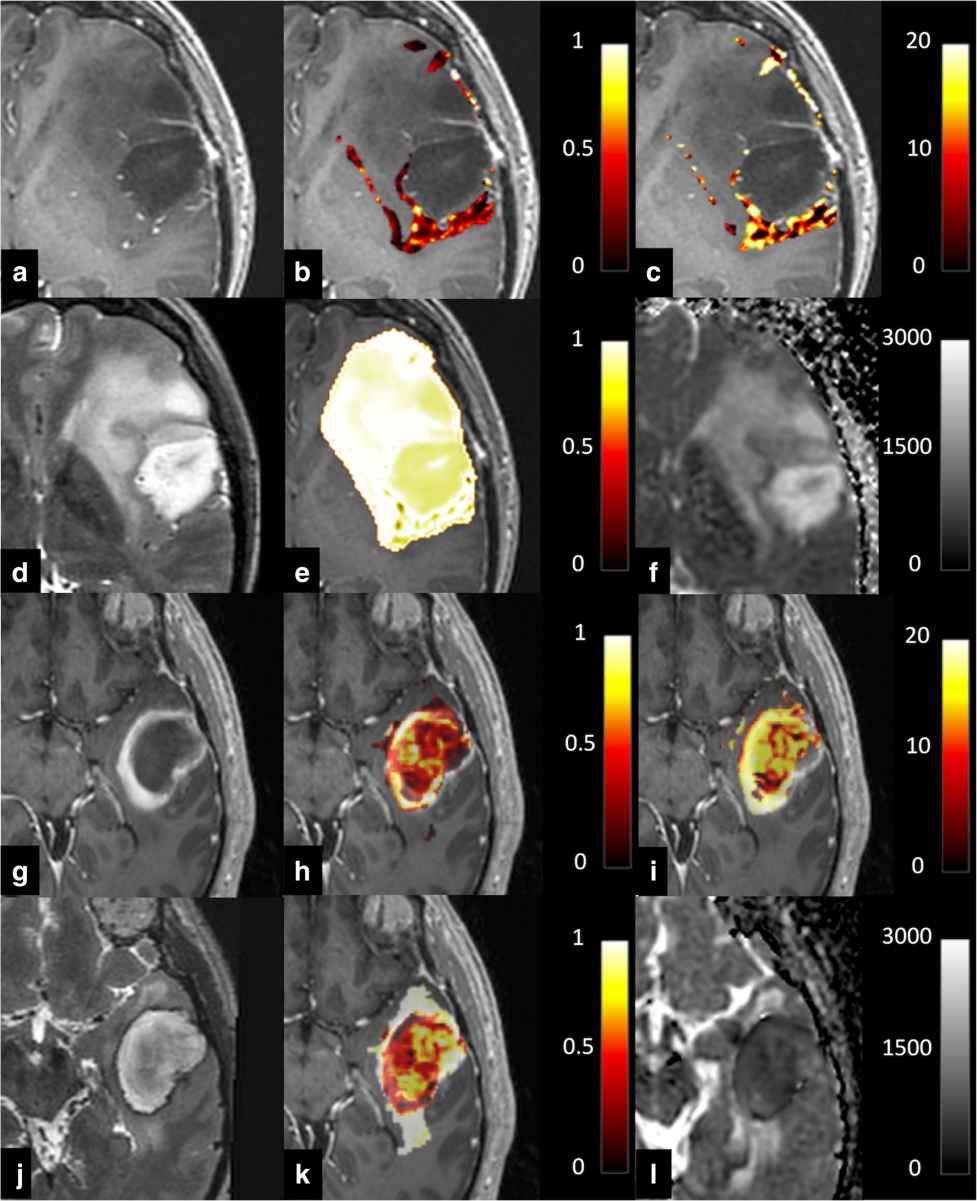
a–f Representative images from a low-grade glioma. a Axial post-gadolinium 3D T1-weighted imaging (T1WI). b Intracellular volume fraction. c Cell radius maps. d Axial T2WI. e Extracellular volume fraction. f ADC map, with a scale of × 10^−6^ mm^2^/s. Colour maps for b, c, e have been superimposed on the greyscale image from a with the colour scale shown for each image. g–l Representative images from a high-grade glioma. g Axial post-gadolinium 3D T1WI. h Intracellular volume fraction. i Cell radius maps. j Axial T2WI. k Extracellular volume fraction. l ADC map, with a scale of × 10^−6^ mm^2^/s. Colour maps for h, i, k have been superimposed on the greyscale image from a with the colour scale shown for each image
